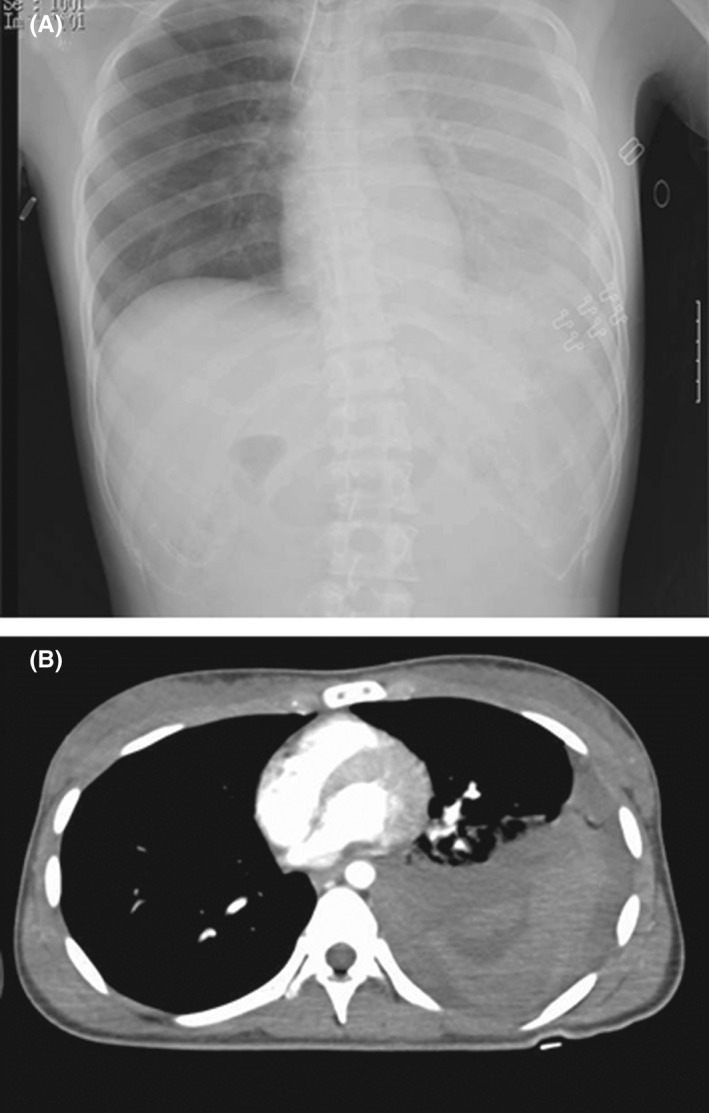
(A) Chest radiography and (B) computed tomography (CT) showing pleural effusion in the left lung
